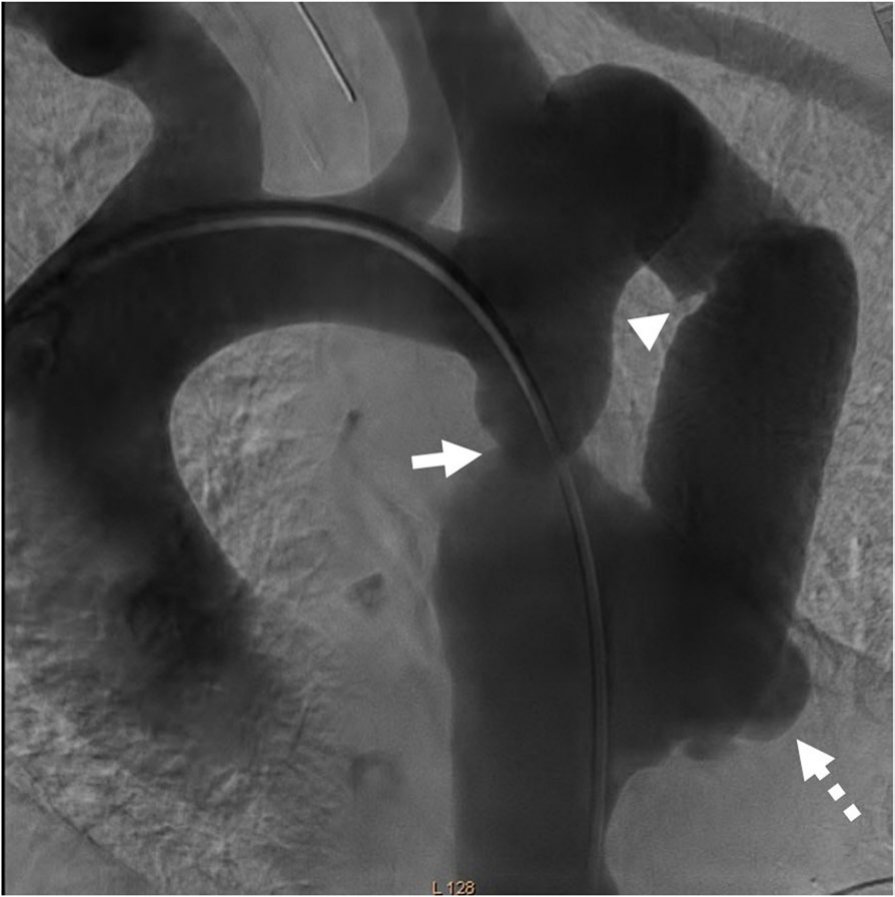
Aortic root injection during fluorosocpy. Confirmed CT angiography results and depicted that proximal anastomotic site emanated from ostioproximal of left subclavian artery. arrowhead: tube graft, arrow: interruption site, dashed arrow: pseudoaneurysm of distal anastomotic site
